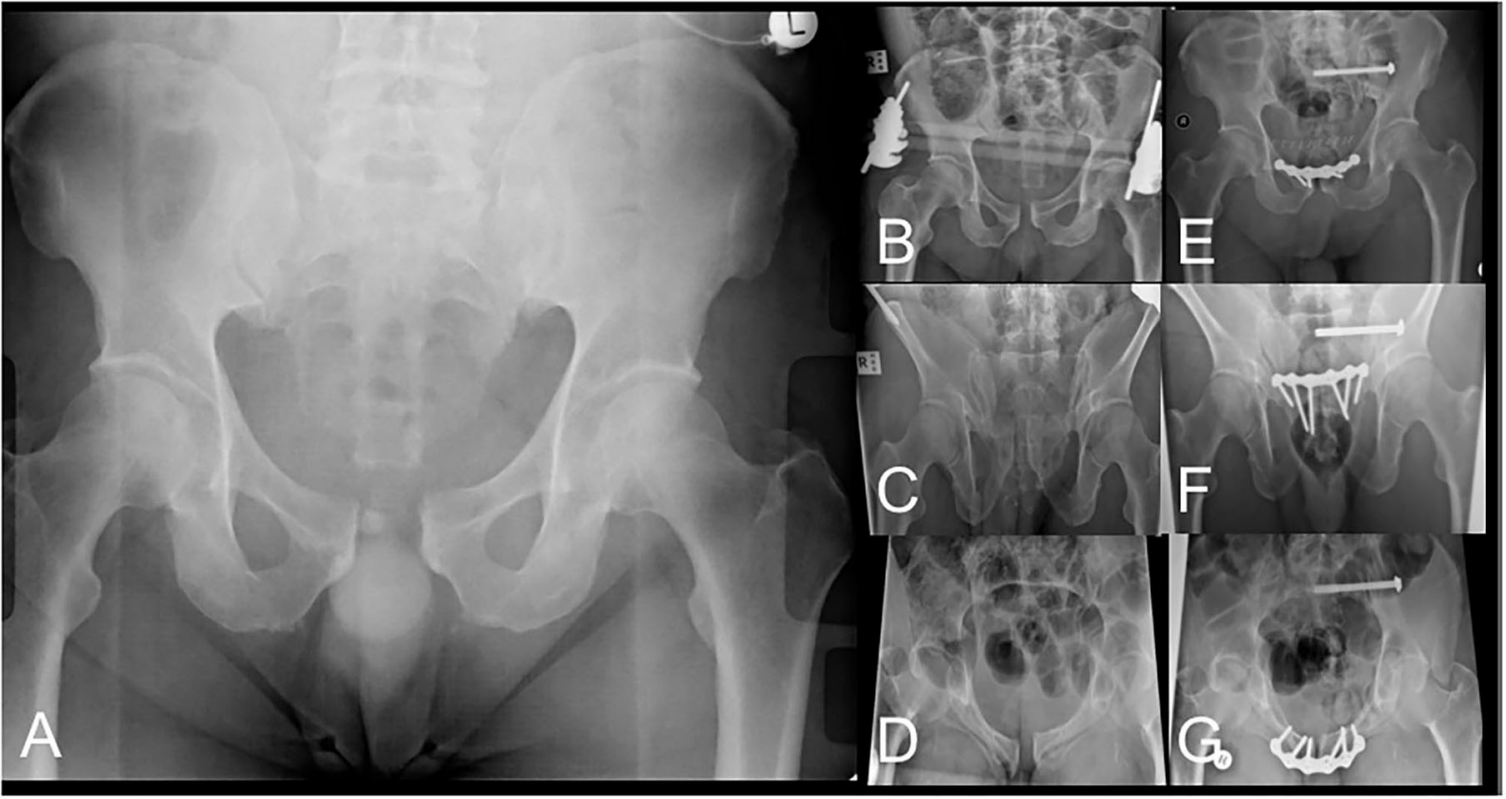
AP pelvis radiograph (A) of pelvic injury classified as left-sided APC2. AP (B), outlet (C) and inlet (D) radiographs after LPEF application demonstrating improved pelvic alignment awaiting definitive surgical management. AP (E), outlet (F) and inlet (G) radiographs after definitive fixation after removal of LPEF.
